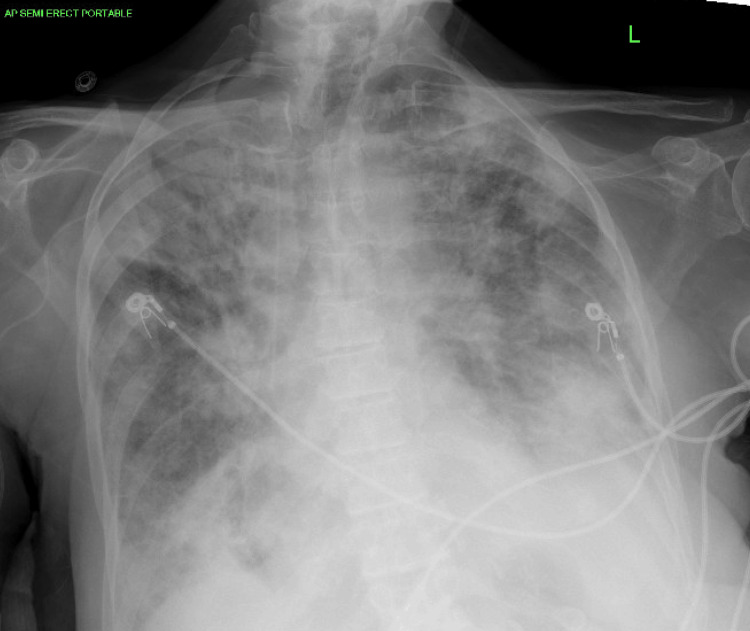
Chest X-ray after five weeks of daptomycin use
